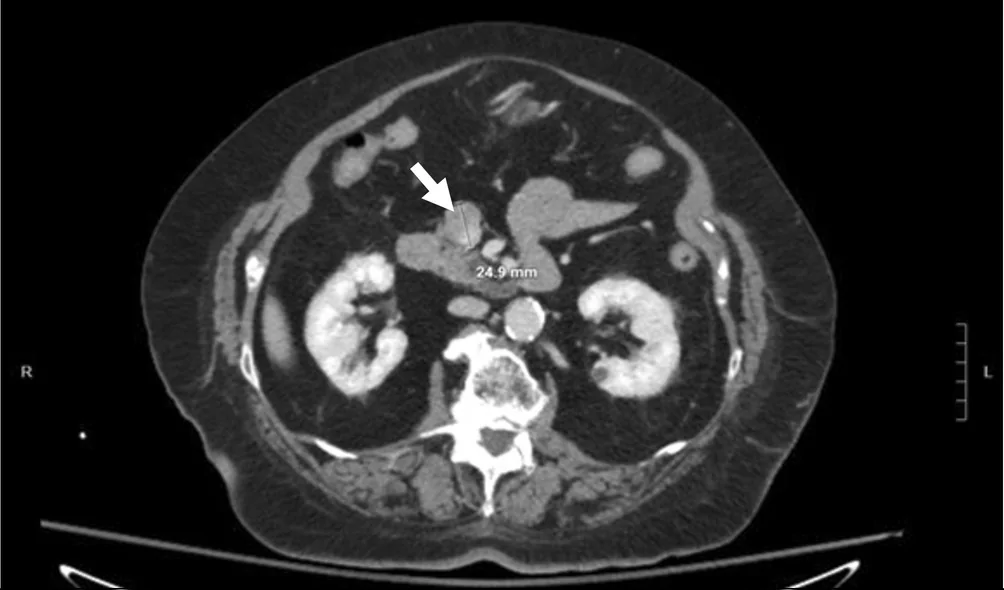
Computed tomography scan abdomen pelvis demonstrating a ~2.5 cm exophytic lesion arising from the pancreatic head (arrow).
